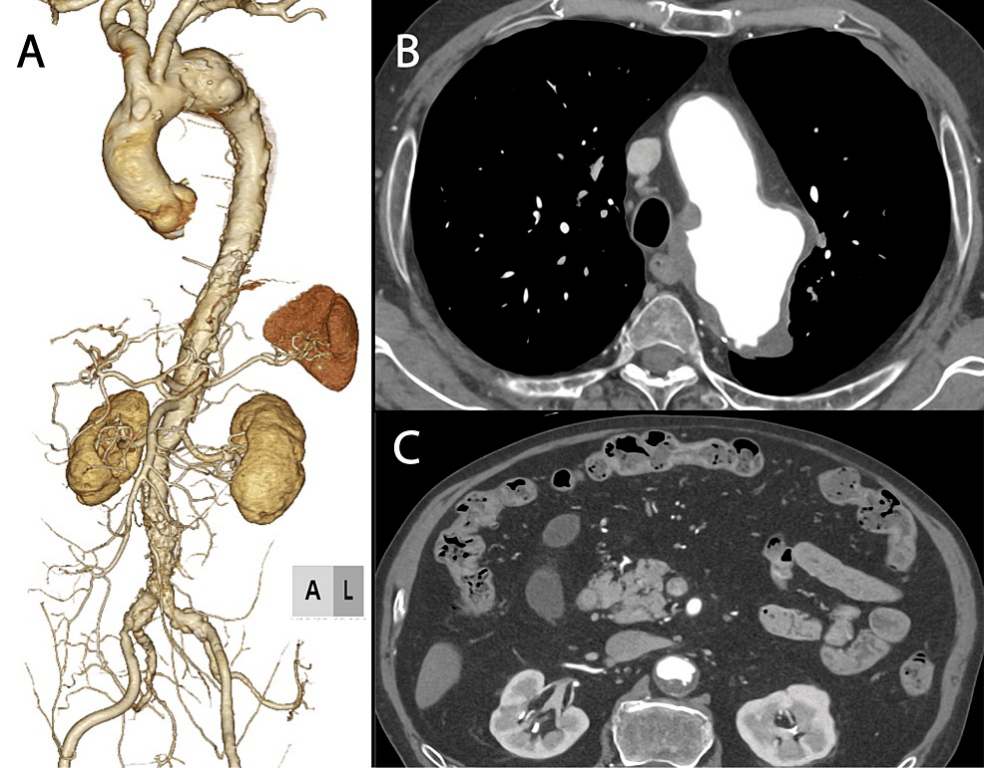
Computed tomography imaging before operation (A) Volume rendering image. The aortic arch aneurysm diameter was 60 mm. (B) Axial image at the aortic arch showed high atherosclerosis changes. (C) Axial image at the pancreas level indicated highly atherosclerotic changes in the aorta and normal pancreas.
